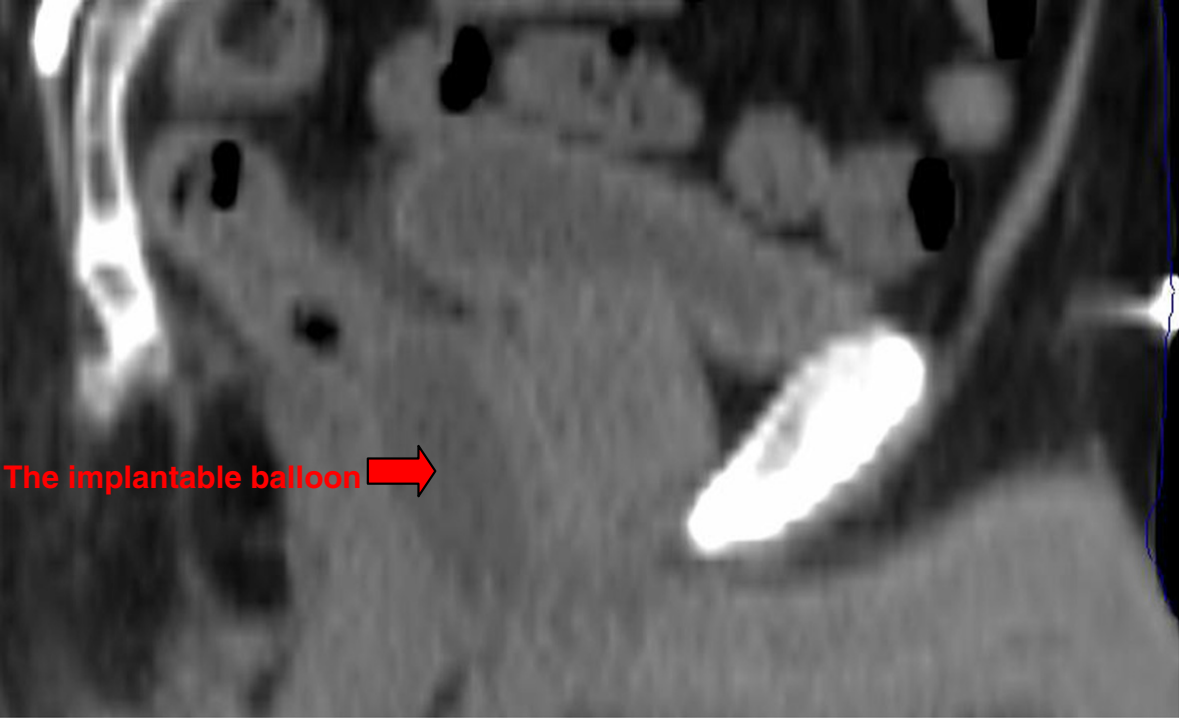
Sagittal view of CT scan, 7 days post balloon implant.
